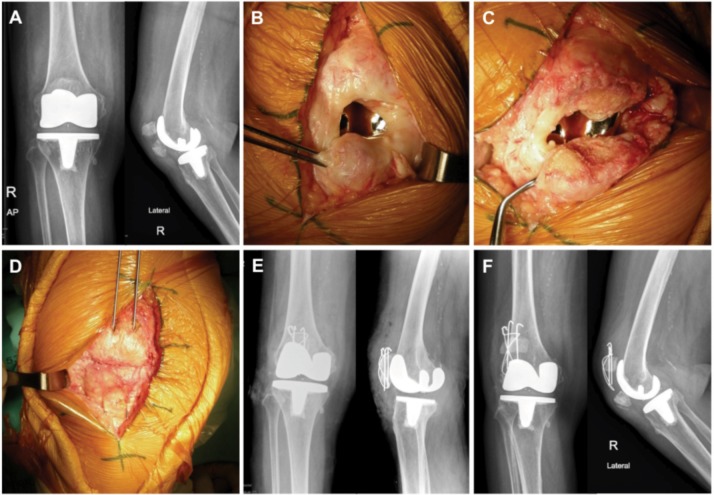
Initial radiographs, intraoperative findings, and postoperative radiographs after the first operation; (A) Preoperative radiographs of right knee revealed transverse periprosthetic patellar fracture with 2-cm fracture displacement. Intraoperative findings showed displaced fracture without callus formation (B), then the fracture bed was prepared (C) and anatomically fixed with tension band wiring (D). Postoperative radiographs on the first day (E) after open reduction and internal fixation with tension band wiring, and one-month postoperative radiographs (F) showed displaced fracture with failed implant fixation.
